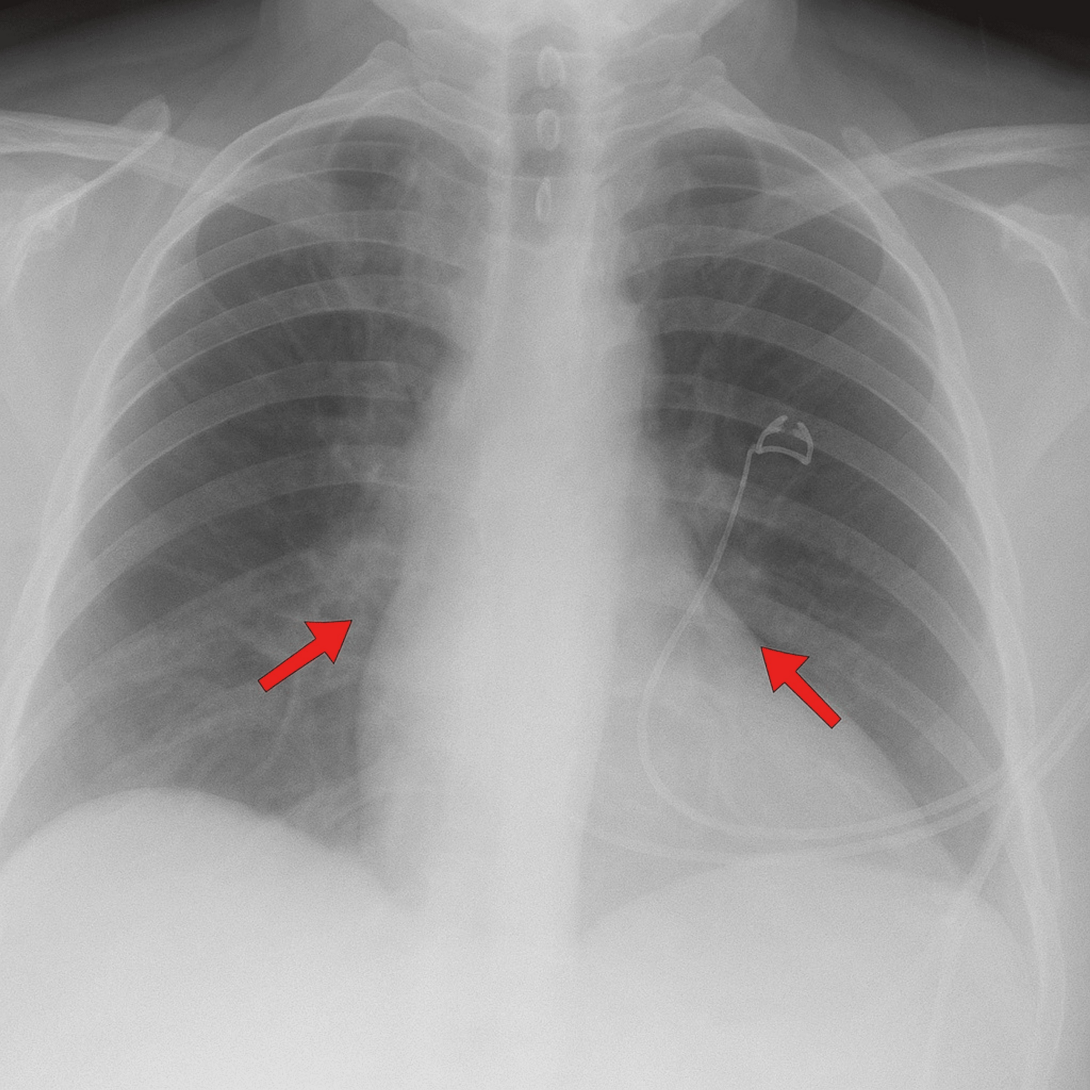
Chest X-ray demonstrating bilateral pulmonary opacities
